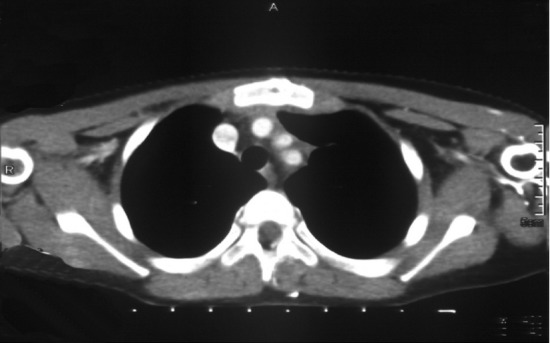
Follow-up CT subsequent to chemotherapy revealing no mass in the mediastinum. CT, computed tomography.
